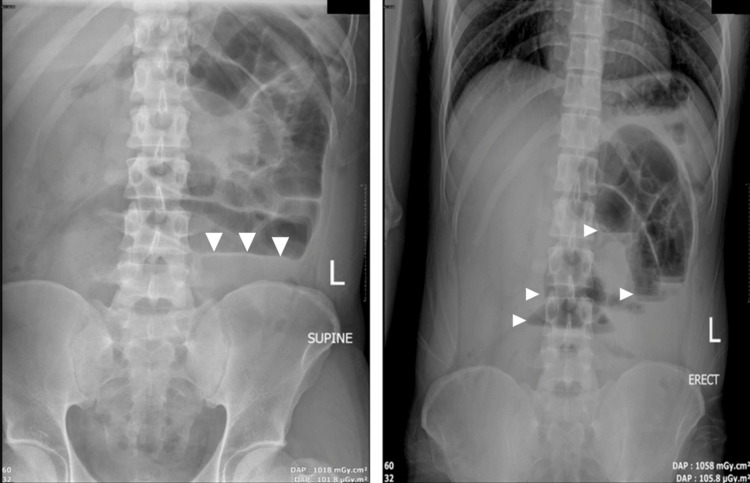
Abdominal X-ray (supine and erect).
